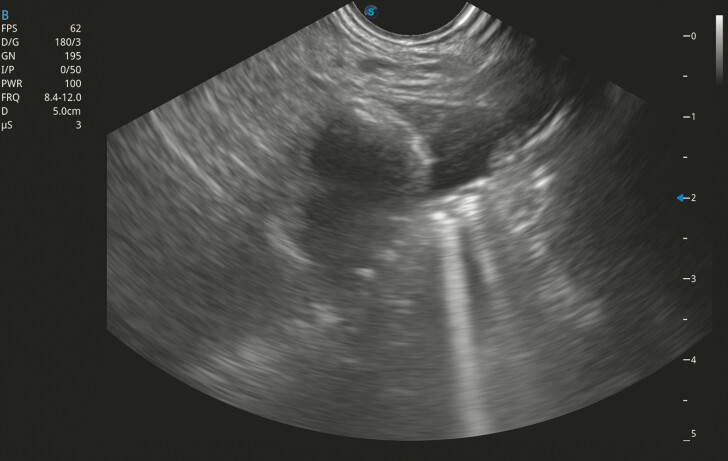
Endoscopic ultrasound visualization showed the dilated jejunum and the scope sign.
